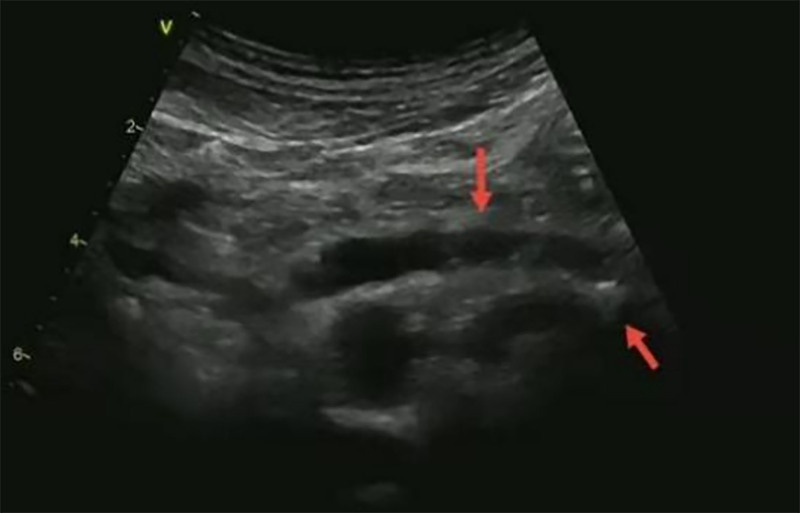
Ultrasound of abdomen showed dilated main pancreatic duct and hyperechoic dots of the pancreatic tail.
